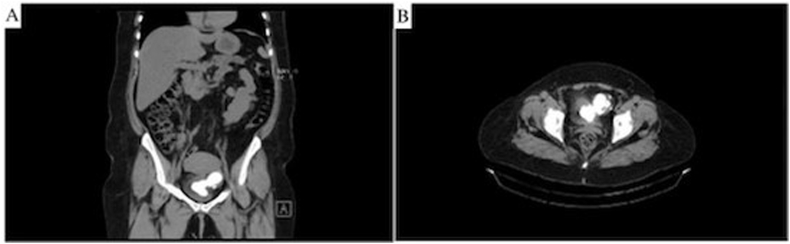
A) Coronal and B) Axial CT scans showing the bi-lobed 10-cm calculus in the bladder and its diverticulum.
